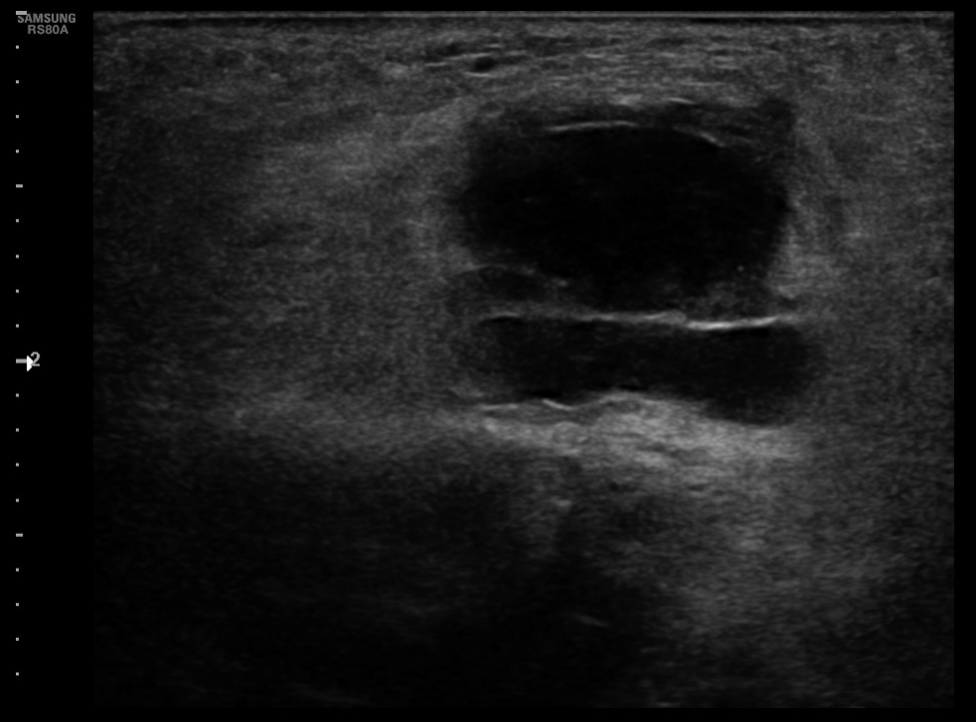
Ultrasound scan of hypoechoic bilobulated mass with heterogeneous content and perilesional edema.
